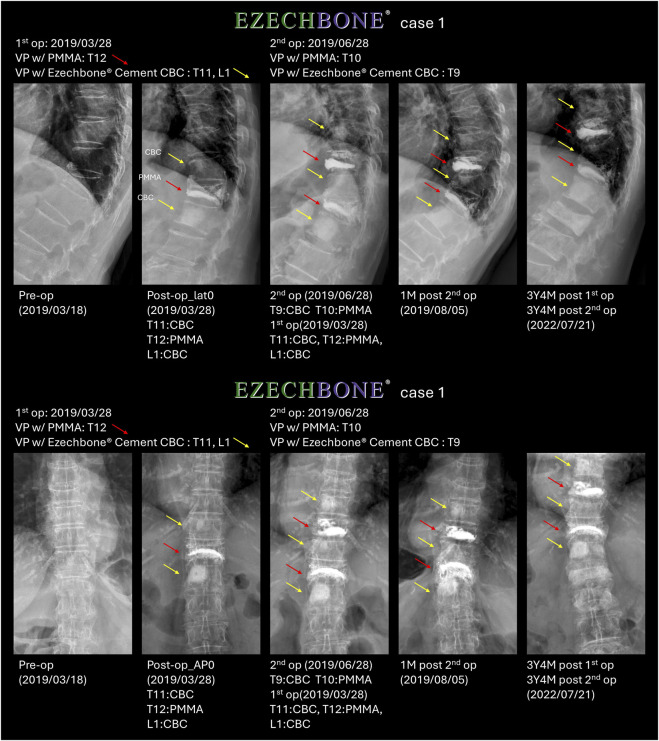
The preoperative and a series of follow-up radiographs in case 1.
